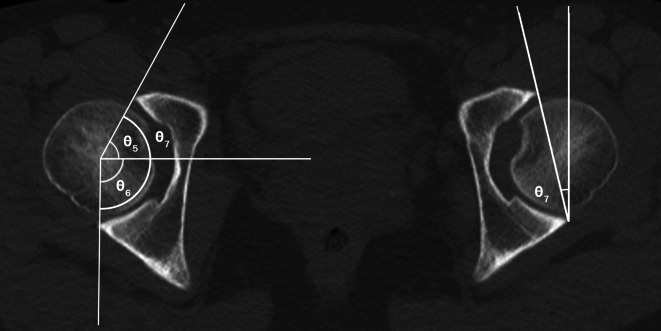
Parameters used to measure acetabular version and axial osseous support. θ5 and θ6 are the anterior acetabular sector angle (AASA) and posterior acetabular sector angle (PASA), respectively. θ7 are the horizontal acetabular sector angle (HASA). θ8 is the acetabular version (AV) described by Anda S. These parameters were measured on were measured on axial images of CT scan at the level of the femoral head center. AASA was measured as the angle between the inter-capital centerline and the line joining the anterior margin of the acetabulum and the femoral head center. PASA was measured as the angle between the inter-capital centerline and the line joining the posterior margin of the acetabulum and femoral head center. HASA was calculated as the summation of AASA (θ5) and PASA (θ6). AV was measured as the angle between the line connecting the anterior and posterior margins of the acetabulum and a line perpendicular to the inter-capital centerline
